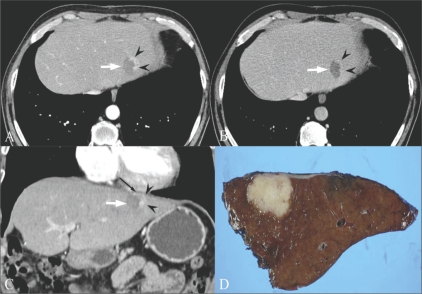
A 55-year-old man was referred due to marginal recurrence of cholangiocarcinoma at the site of a previous radiofrequency ablation. Arterial phase (A) and portal venous phase (B) axial CT scans show a marginally enhancing lesion (arrowheads in A) with wash-out (arrowheads in B) at the periphery of the previously ablated site (arrow) in the lateral segment of the left lobe of the liver. Initially, repeat radiofrequency ablation was considered for this recurrent mass. However, the coronal MPR image (C) revealed close proximity between the suspected viable tumor (arrowheads) and the inferior pericardium (thin black arrow). To avoid thermal damage to the pericardium and to guarantee a safe and clear margin from the viable tumor, the patient underwent surgery. The lesion was separated from the pericardium and pathologically proven to be a recurrent cholangiocarcinoma. This case demonstrates the role of MPR images as a guidance to determine which procedure is appropriate. MPR : multiplanar reconstruction
